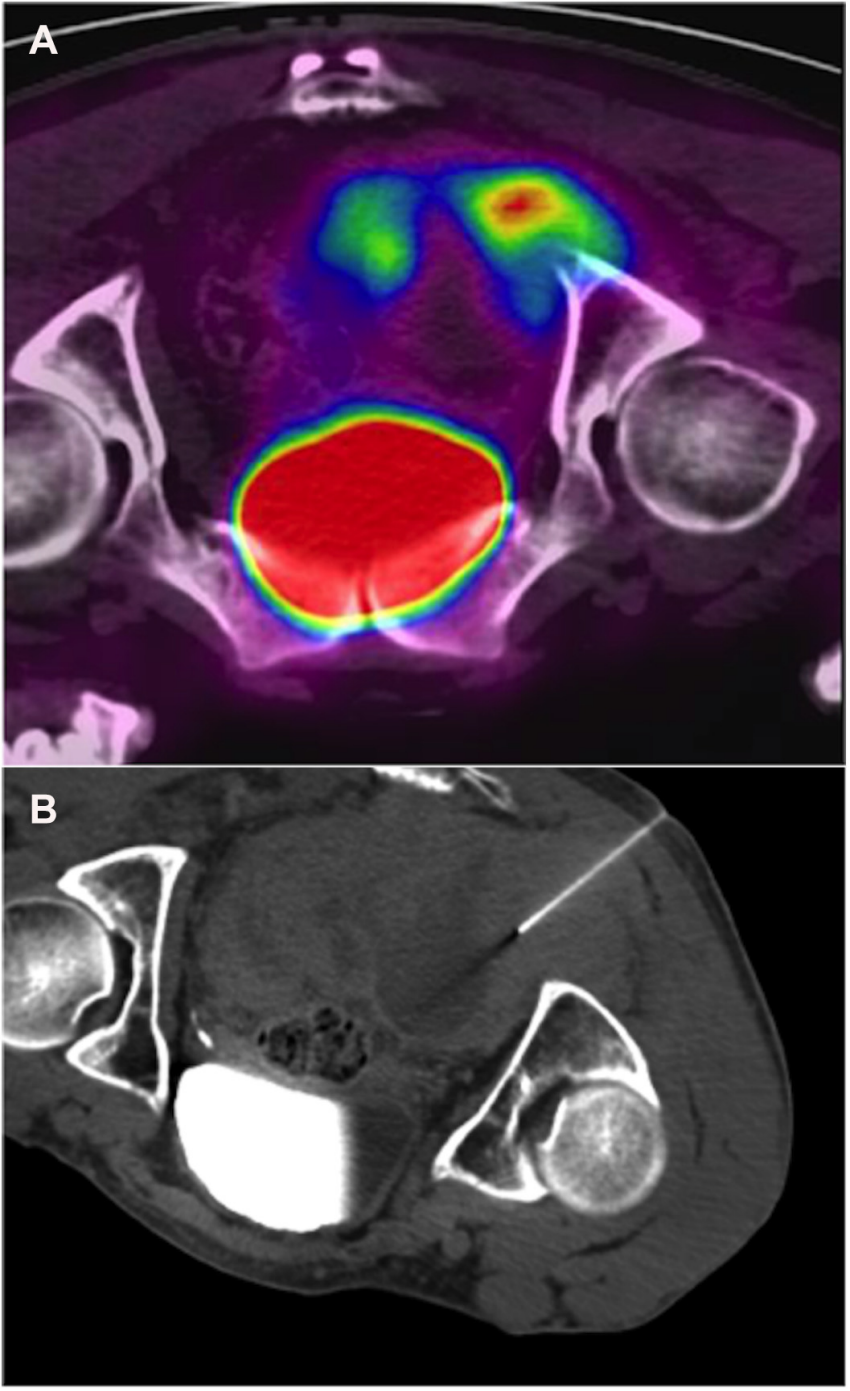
PET/CT-guided percutaneous biopsy of a metabolically active pelvic soft-tissue tumor in 34-year-old man with NF1. (a) Initial PET/CT discriminate FDG-avid portion at the right sciatic notch. (b) The needle is shown to be in most metabolically active portion of FDG-avid mass. Pathology results concluded to intermediate-grade MPNST. The tumor was unresectable and the patient was treated by chemotherapy (adriamycin and ifosfamide). He died 17 months after the diagnosis.
